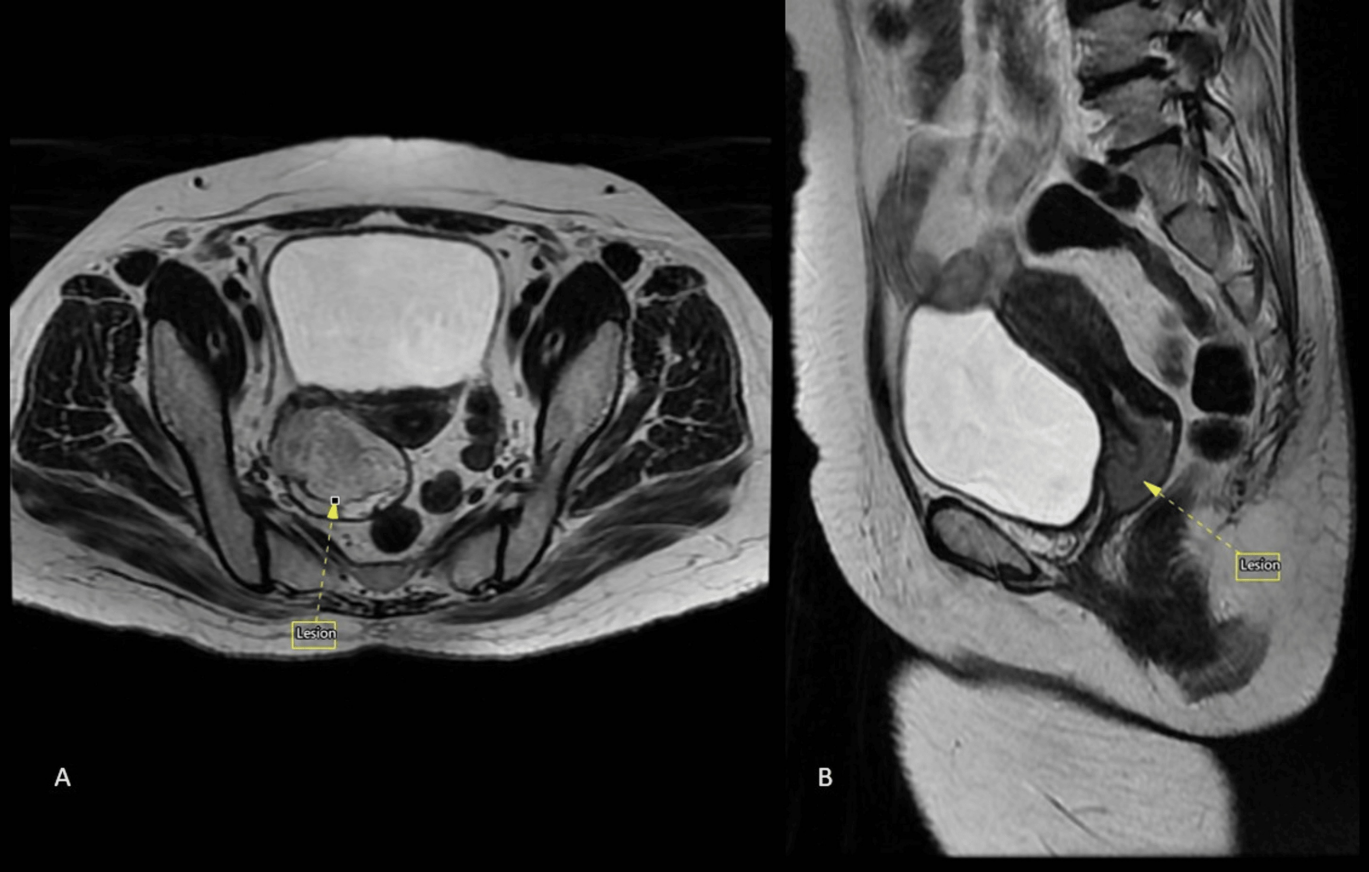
Magnetic resonance imaging (MRI) T2 axial (A) and sagittal (B) sections at the level of vagina shows a well-defined heterogeneous T2 hyperintense lesion originating from the posterior vaginal wall
